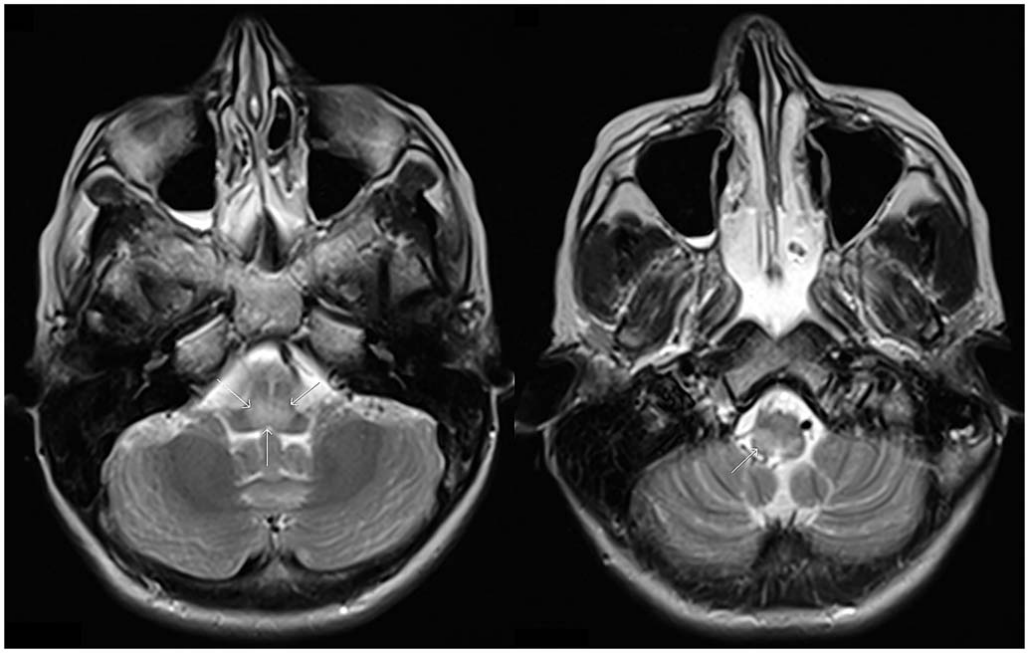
MRI brain on day 6 of admission. Axial T2-weighted images in a patient with rabies due to Duvenhage virus infection, at day 6 after admission; hyperintense signal in the posterior part of the medulla oblongata (arrow).
